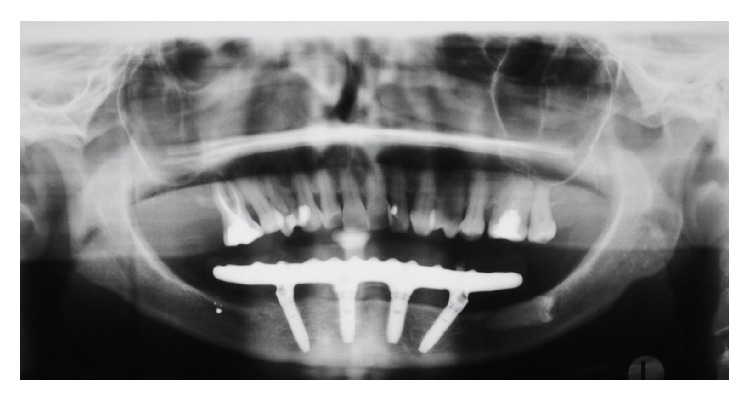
Orthopantomography of the patient 6 months after surgery.
